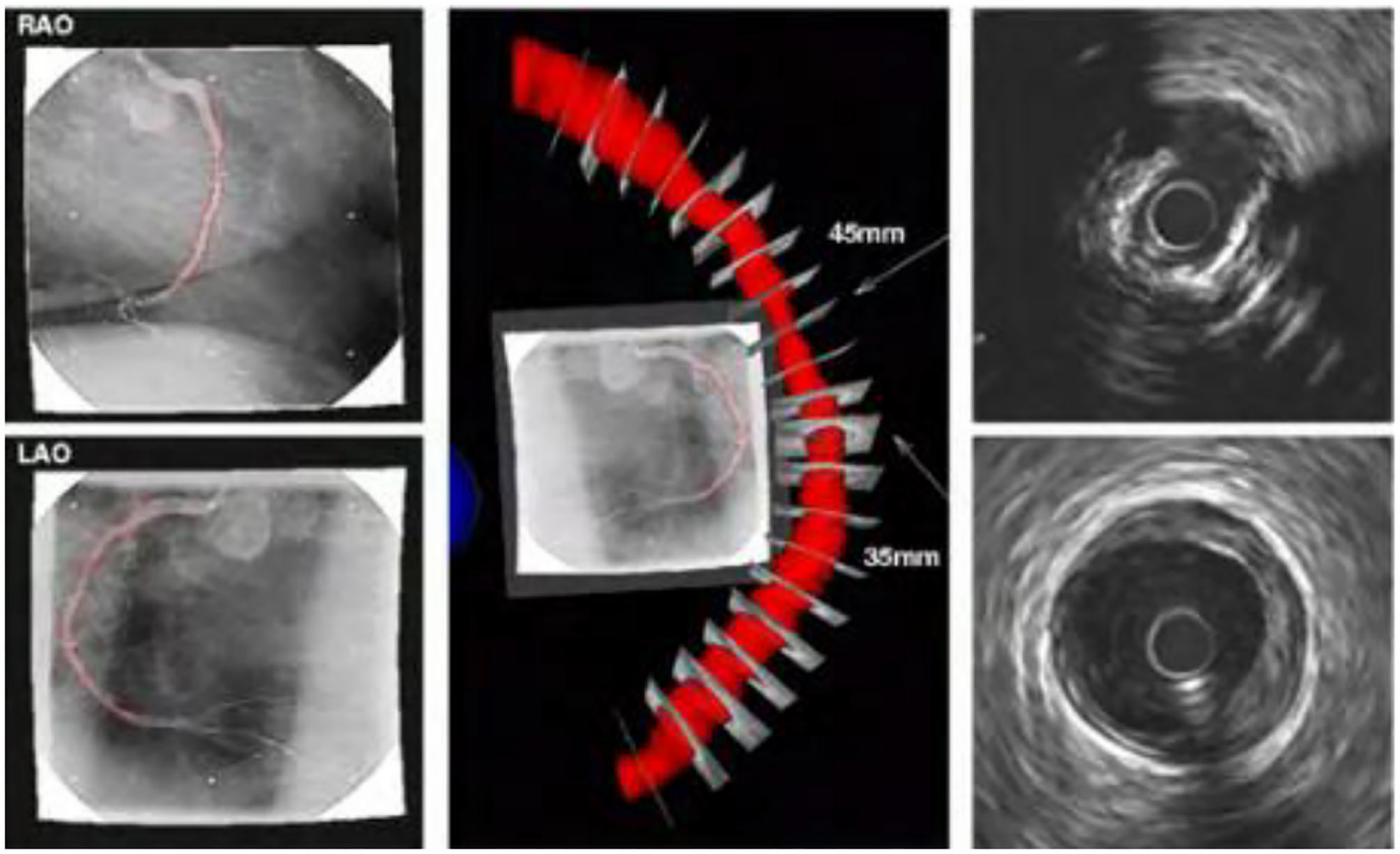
Reconstruction results of new type of intravascular ultrasound combined with X-ray angiography.
